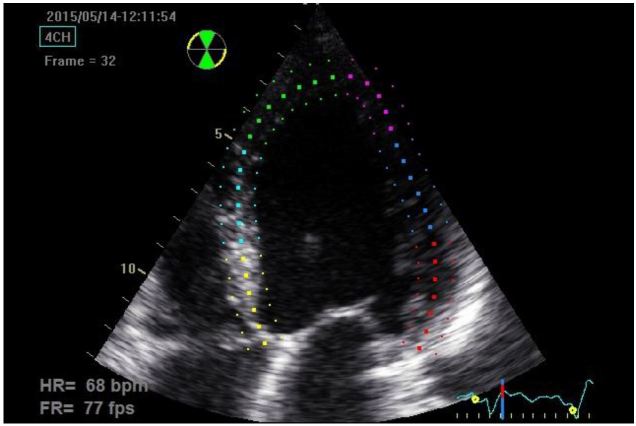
Apical 4-chamber image demonstrating semi-automated tracing of the endocardial border using two-dimensional speckle-tracking software. Depth is minimized excluding part of the left atrium in order to maximize frame rate.
